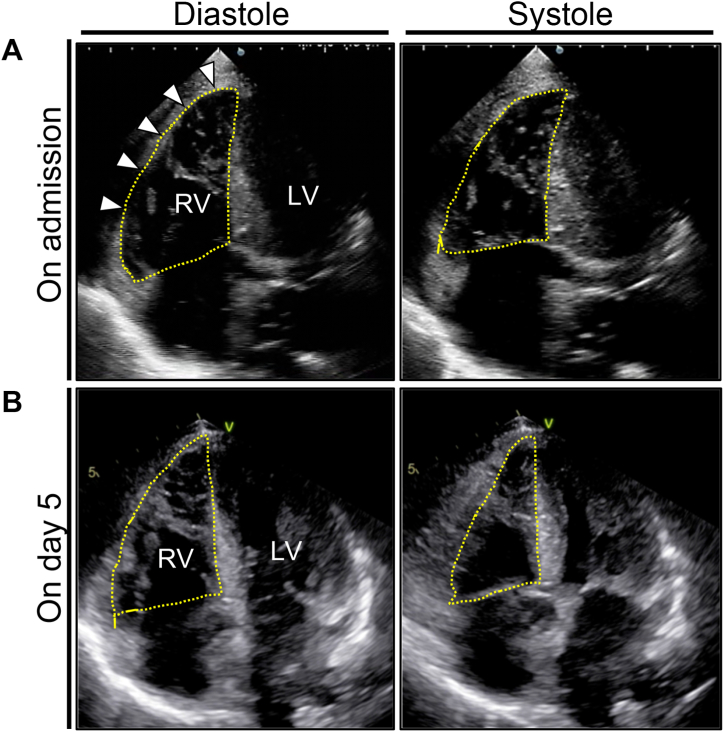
Serial echocardiography (A, on admission; B, on day 5) Apical four-chamber views of the heart during diastole and systole. Note the severely reduced systolic function of the apico-mid right ventricular free wall with right ventricular enlargement (arrowheads). LV, left ventricle; RV, right ventricle.
